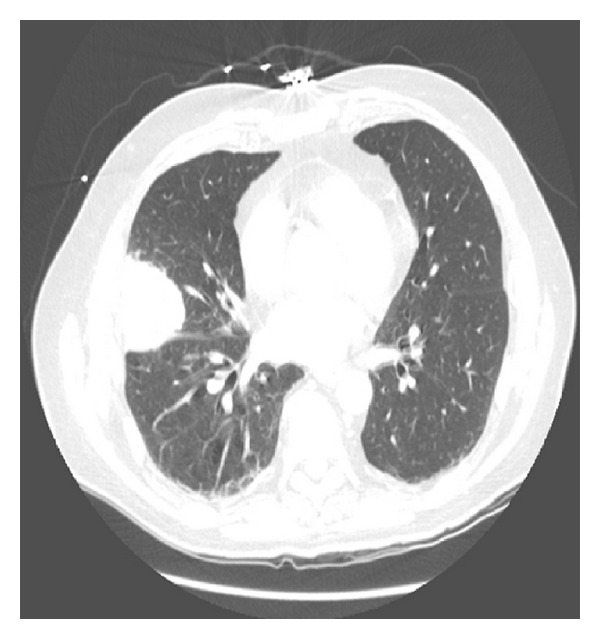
Chest CT scan demonstrating a large, rounded mass in the periphery of the right middle lobe.
